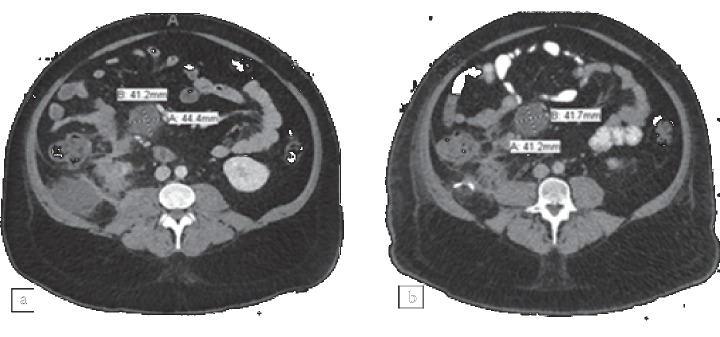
Computed tomography scan before starting antimicrobial therapy in Patient 3 shows a locule of complex fluid in the midline near the superior mesenteric vein measuring 4.4 × 4.1 cm (a). Computed tomography at time of antimicrobial completion shows a persistent phlegmonous collection measuring 4.2 × 4.1 cm (b).
